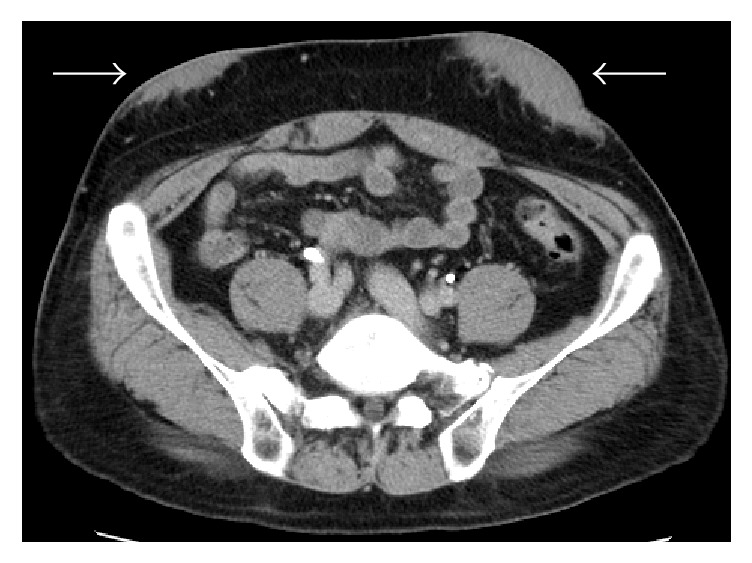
Contrast-enhanced CT of the abdomen (equilibrium phase): in the left and right subumbilical regions, subcutaneous masses with irregular margins are observed, each measuring approximately 60 mm. The density is heterogeneous and higher than that of the surrounding adipose tissue, without contrast-enhancement effect (indicated by arrows).
